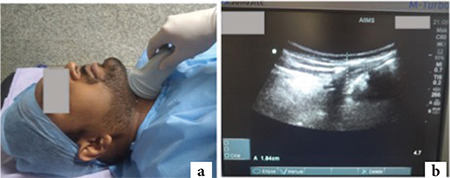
a) Third step Airway-USG-patient head in neutral position with curvilinear transducer placed in Infrahyoid region at the midline in sagittal plane (Note the transducer is moved caudally from step-1 to trace the epiglottis still keeping the hyoid bone in plane). b) Ultrasonographic image at third step. A=Distance from skin to posterior surface of epiglottis, measured just below hyoid bone (Note Hyperechoic air-mucosa interface at posterior surface of epiglottis). USG, ultrasonography.
